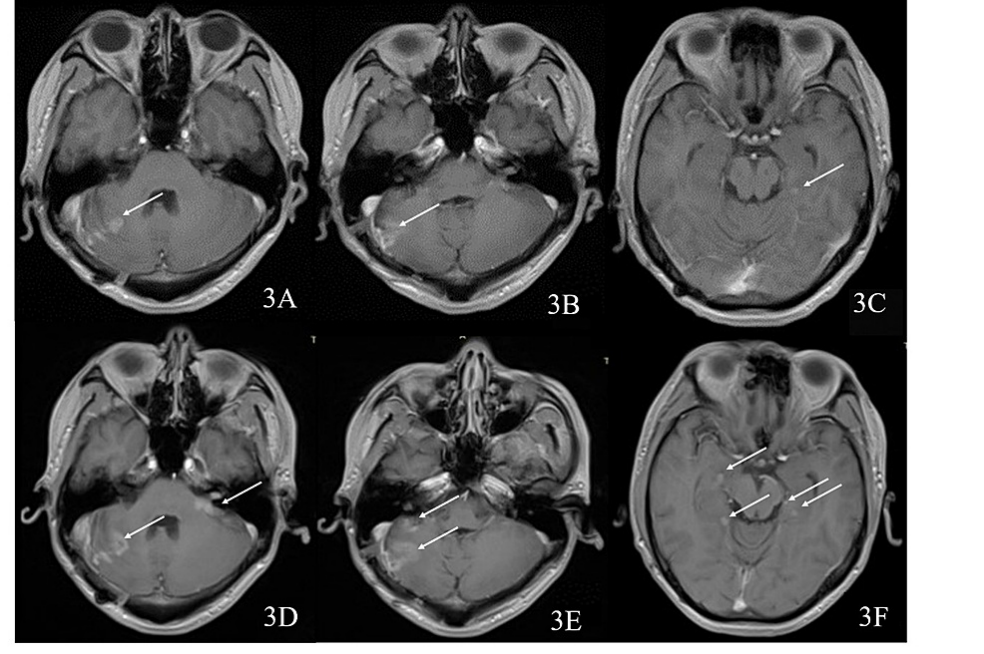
Postoperative MRI scans At 15 months after the operation, a magnetic resonance imaging (MRI) scan showing patchy enhancement in the right cerebellar hemisphere, which is adjacent to meningeal enhancement (3A-3C), and punctate enhancement signal in the left hippocampus. MRI at 17 months postoperative showing the lesions in the right cerebellar hemisphere to be more extensive than before, and the newly developed left brachium pontis, left cerebellopontine angle area, left auditory nerve trunk, and bilateral hippocampus areas to be abnormally enhanced (3D-3F).
